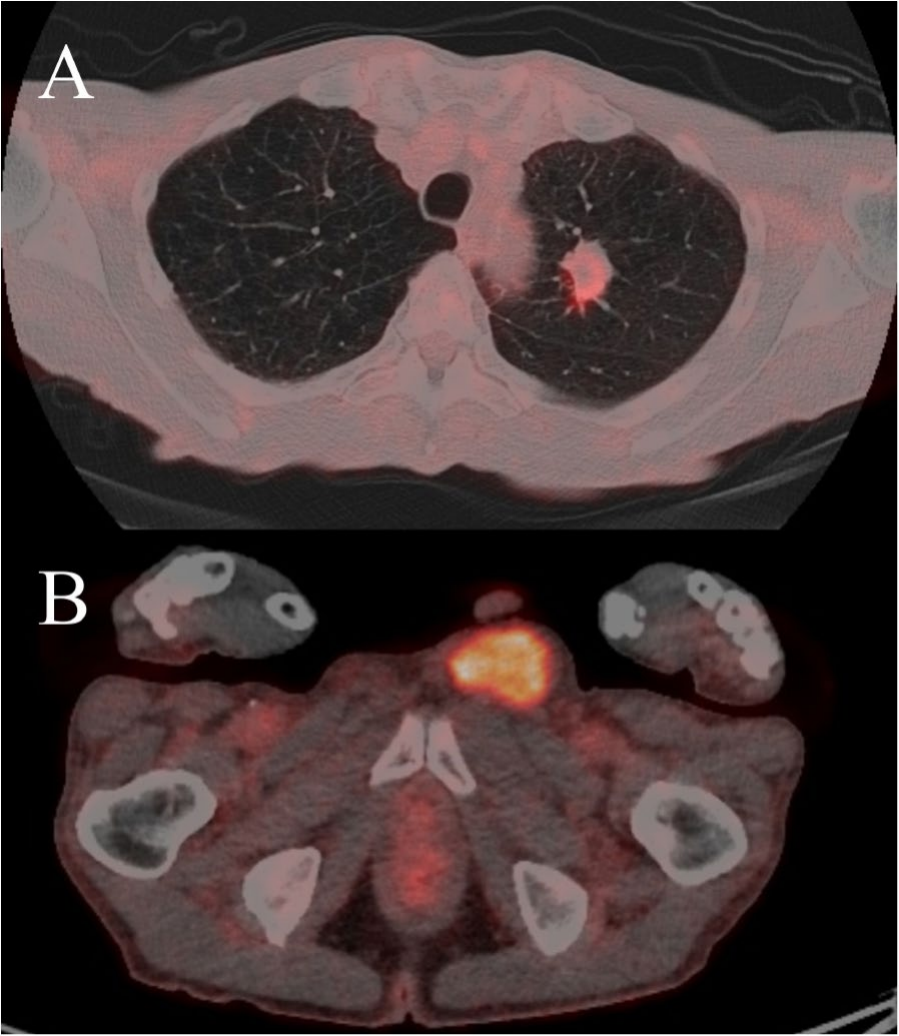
Positron emission tomography–computed tomography findings of the two sarcomas. A scan shows a high accumulation of both the pulmonary sarcoma (SUVmax, 3.5) (A), and inguinal dedifferentiated liposarcoma (SUVmax, 7.7) (B), with no other abnormal accumulation. SUVmax, maximum standard uptake value
